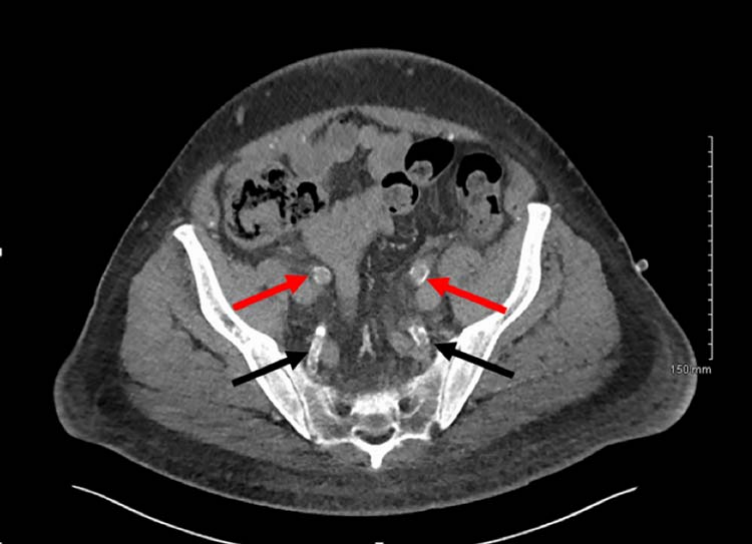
Axial slice of a non-contrast CT AP with red arrows showing calcified external iliac arteries and black arrows showing calcified internal iliac arteries.
